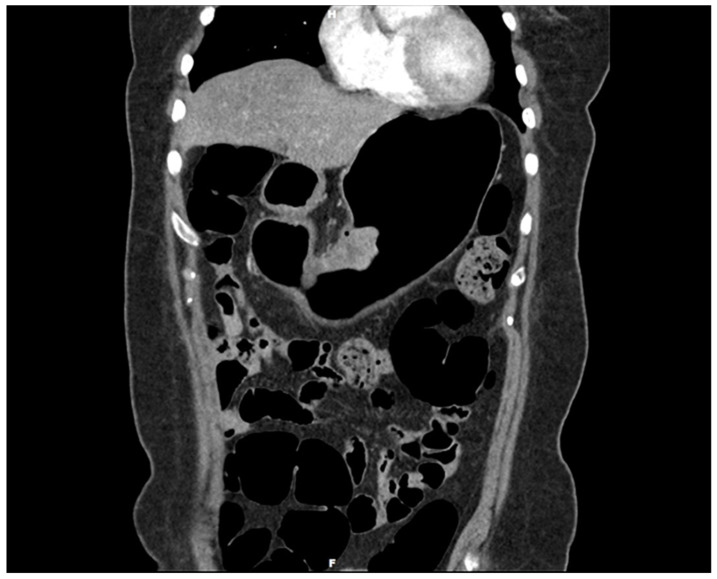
Gastroscopy with three-dimensionally enhanced computed tomography (3D ECT) showing a protruding tumor in the mid-body on the lesser curvature. Gastric duplication cysts (GDCs) are rare congenital anomalies first described in 1911 by Wendel and typically identified in infancy or childhood. Although the exact pathogenesis remains elusive, these cysts are believed to result from aberrations during embryonic development of the foregut [1,2,3]. Characteristically, they share a common wall with the stomach and possess an epithelial lining similar to the gastrointestinal tract [4]. The clinical presentation of GDCs can vary significantly, ranging from asymptomatic to severe abdominal symptoms, depending on their size, location, and the presence of associated complications. Although most GDCs are benign, there have been sporadic reports of malignant transformations within these cysts. Adenocarcinoma is the most commonly reported malignancy arising from GDCs, as evidenced by case reports and small case series [5,6,7,8,9]. Moreover, mixed tumors arising from GDCs are rare. To date, only two cases of mixed adenocarcinoma and squamous cell carcinoma arising from GDCs have been reported [10,11]. Herein, we describe a rare case of mixed adenocarcinoma and squamous cell carcinoma that developed within a GDC. However, it differs from the previous report in that the adenocarcinoma component was pancreatobiliary-type ductal adenocarcinoma. Moreover, this case report is the first to report a mixed pancreatobiliary ductal adenocarcinoma and squamous cell carcinoma in a GDC. It contributes a novel aspect to the scientific understanding of the malignant potential of GDCs and underscores the necessity for vigilance in managing these rare entities. By examining this case, we aim to enrich the discourse on GDCs, particularly their neoplastic capabilities, and provide insights into more effective diagnostic and therapeutic strategies. A 54-year-old Korean woman presented with melena that began a month ago and vomiting with hematemesis over the last 3 days. The patient had no other medical, surgical, or significant personal or family histories. She visited a local hospital for assessment and underwent gastroscopy. Gastroscopy revealed a protruding mass with bleeding in the stomach, which was suggestive of malignancy. The local hospital could not perform a biopsy due to the bleeding, and the patient visited our hospital for further evaluation. A 3D ECT scan revealed a protruding mass in the lower gastric body and enlarged perigastric lymph nodes (this figure). An esophagogastroduodenoscopy revealed an approximately 5 cm-sized protruding mass in the mid-body of the lesser curvature. Endoscopic biopsy revealed a malignancy suggestive of mixed adenocarcinoma and squamous cell carcinoma. PET-CT detected a 5 cm-sized focal hypermetabolic activity in the body of the stomach and focal increased fluorodeoxyglucose uptake in the perigastric lymph nodes; otherwise, the findings were unremarkable. Upon laboratory examination, the results of the complete blood count and routine biochemical investigations were unremarkable. Blood tumor marker tests showed elevated levels of carcinoembryonic antigen (CEA) (52.2 ng/mL; normal range: 0.0–3.4 ng/mL) and carbohydrate antigen 19-9 (CA19-9) (317.0 U/mL; normal range: 0–34 U/mL).
